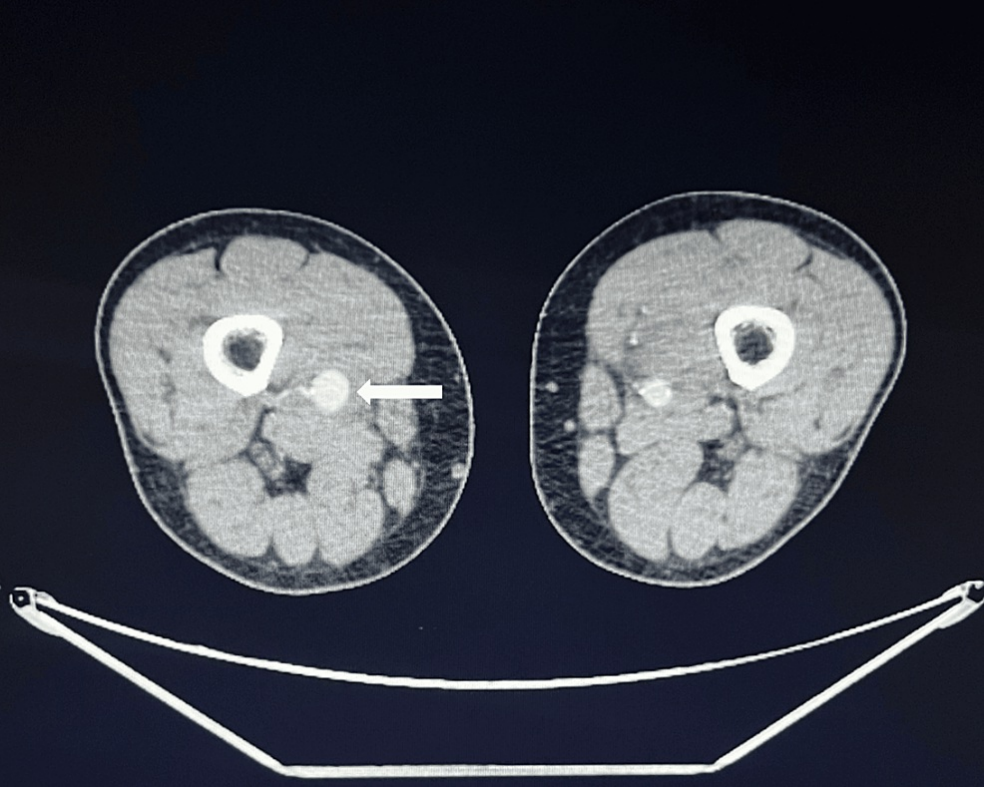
CTA revealing the right-sided superficial artery aneurysm with dimensions of 2.5x3.5cm. CTA: Computed tomography angiography
